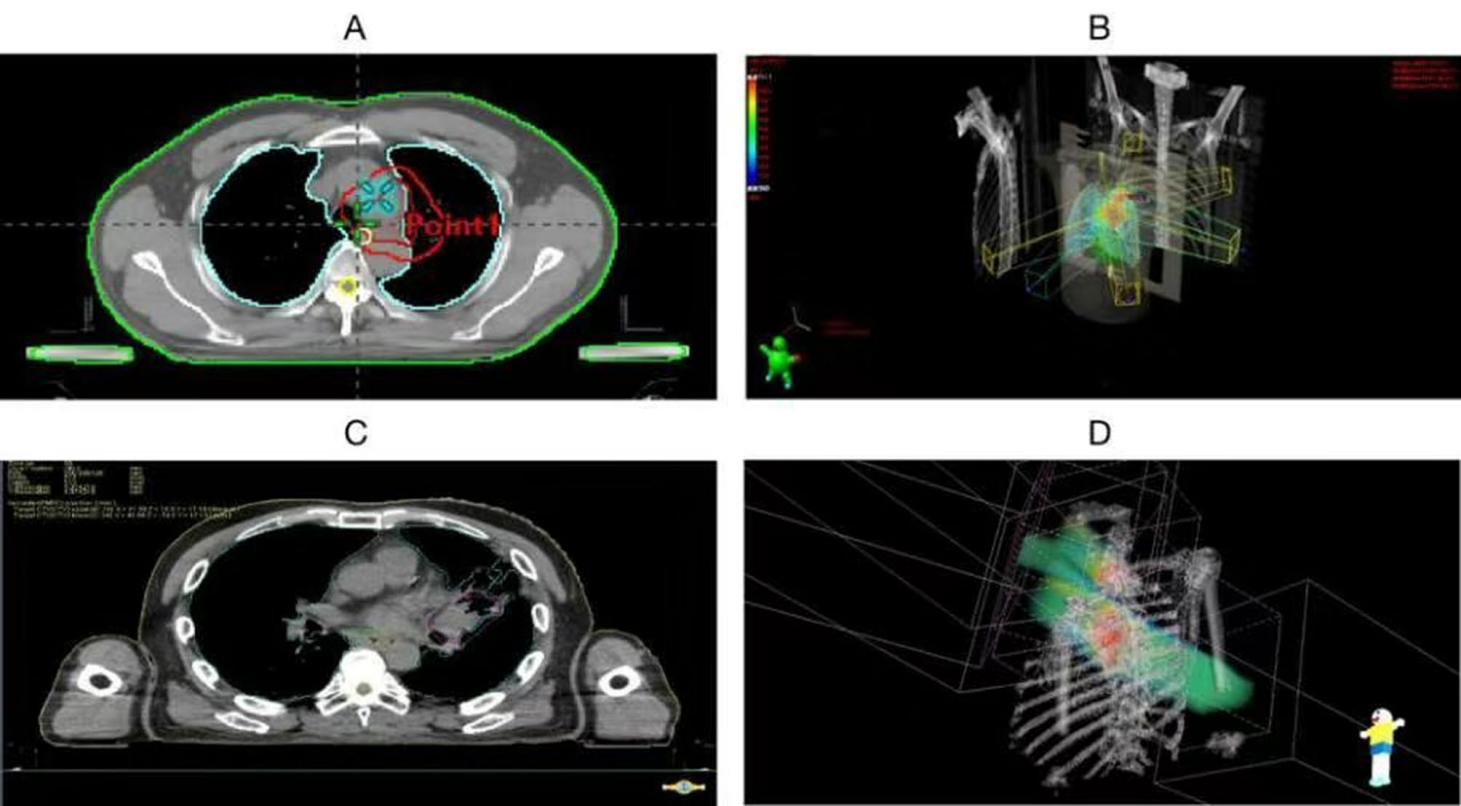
Radiotherapy Target Area and Field. Note: A and B represent the target area and field distribution for intensity-modulated radiation therapy (IMRT) with X-rays, respectively; C and D represent the target area and field distribution for fixed-angle proton therapy, respectively
